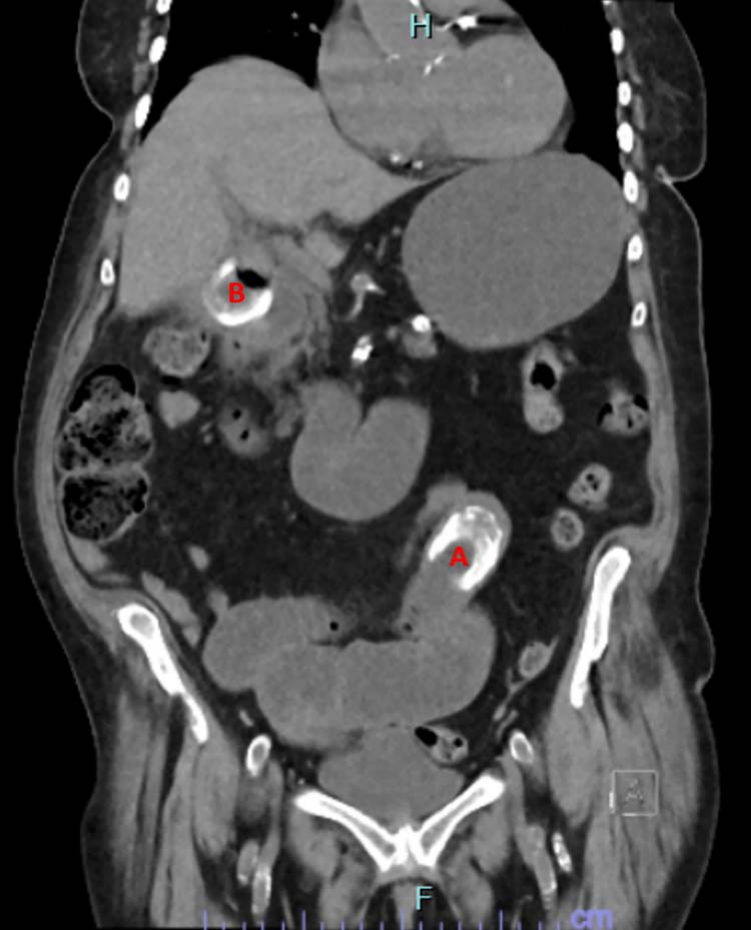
CT image showing obstructing gallstone (A) and gallstone in cholecystoduodenal fistula traversing duodenal wall (B).
